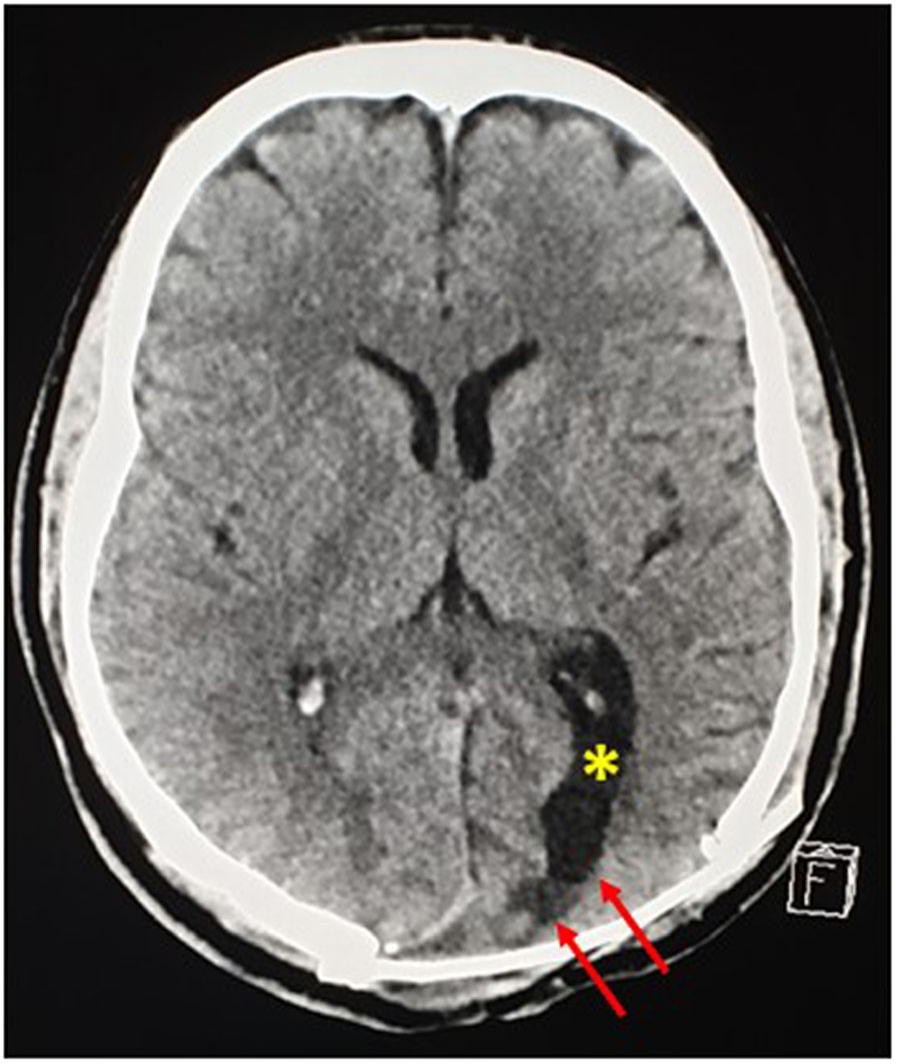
A Computed tomography scan post excision of the left occipital diploic lesion showed atrophic left occipital lobe with encephalomalacia (Red arrows), and ex-vacuo dilatation of the occipital horn of the left lateral ventricle (Yellow asterisk)
